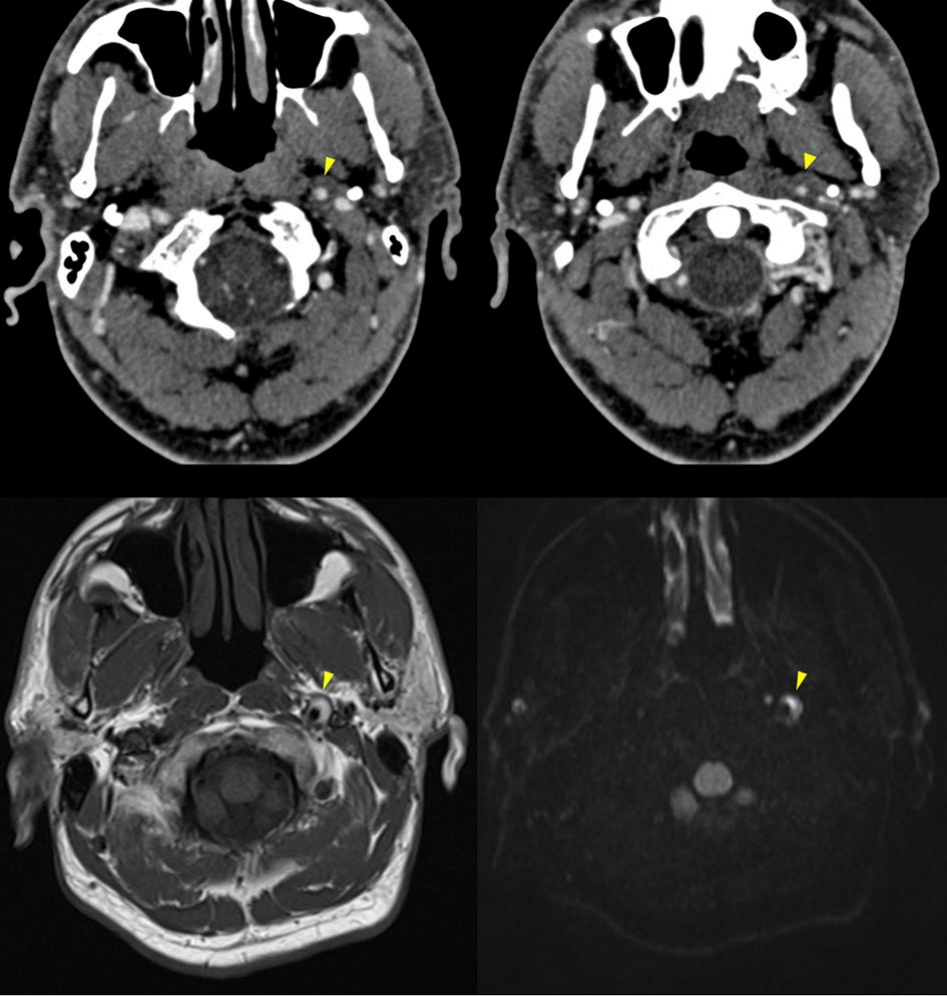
CTA and MRI findings of arterial dissection in the presence of a mural hematoma. CTA (top row) shows a slight lumen reduction of the distal cervical segment of the left internal carotid artery, where an intramural hematoma is also suspected, showing isodensity with the surrounding soft tissues (arrowheads). MRI (bottom row) at the same level more clearly depicts a “crescent sign” consistent with a subacute intramural hematoma on T1-weighted (bottom left) and on DWI (bottom right) sequences (arrowheads)
